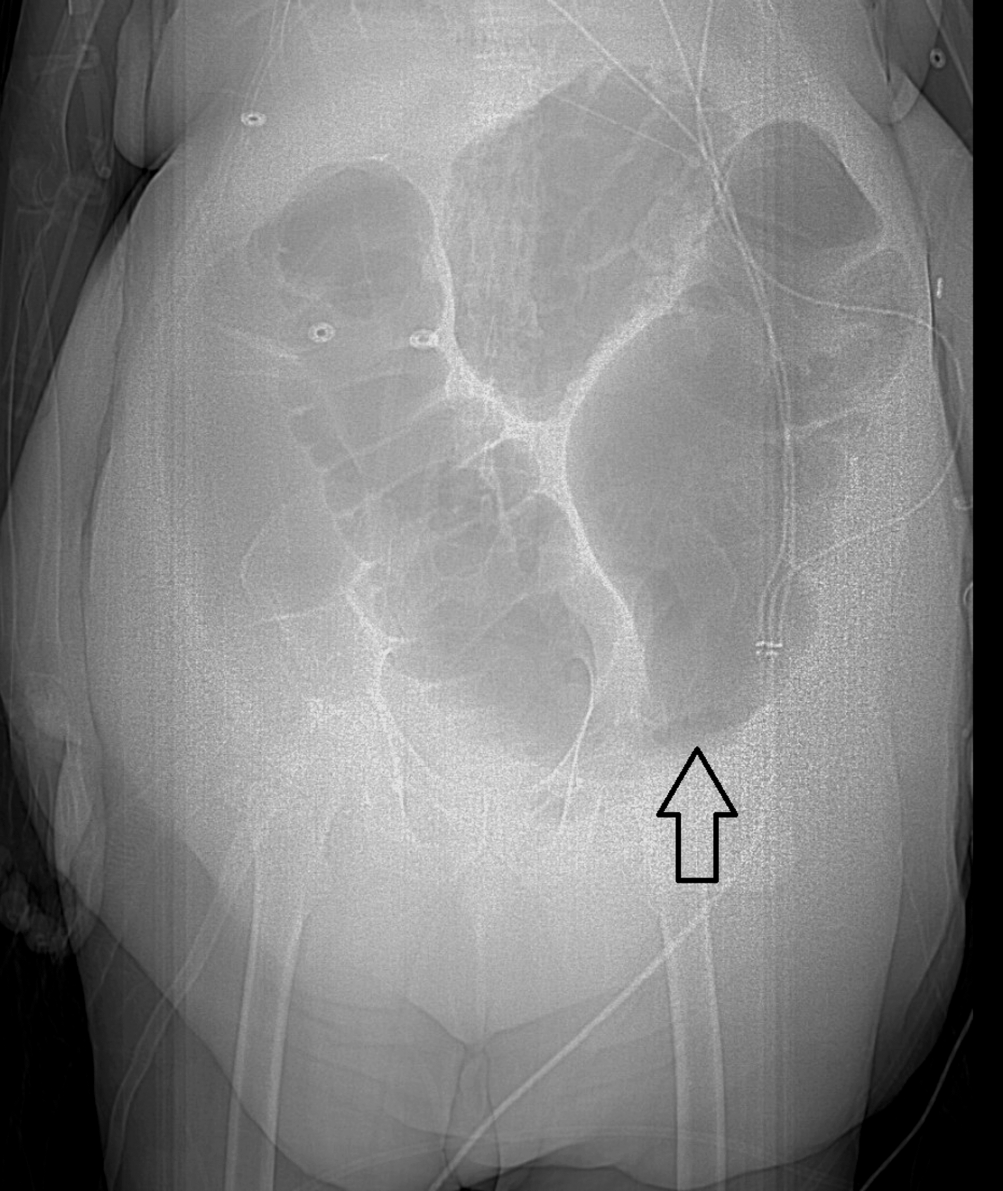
An upright abdominal X-ray showing colonic dilatation (arrow)
